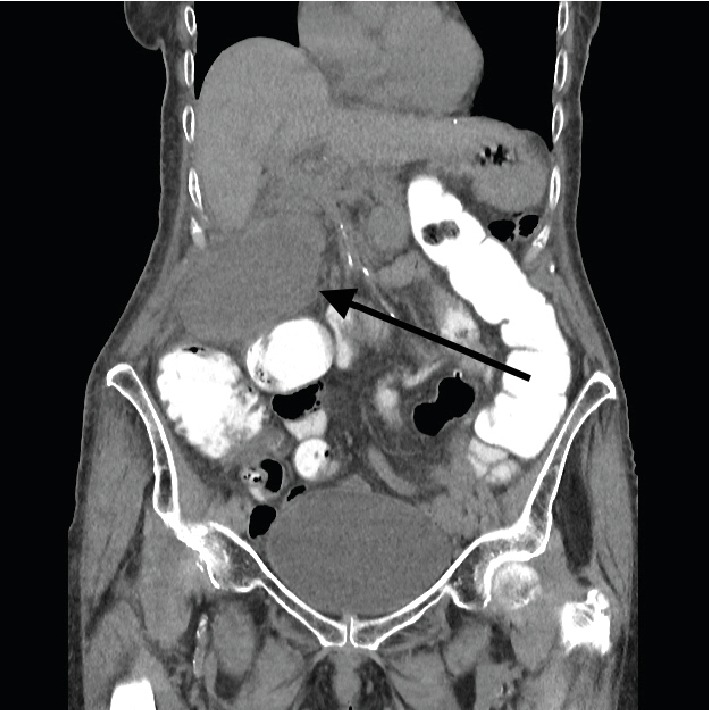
Coronal nonenhanced CT image showing a markedly distended gallbladder (black arrow) and fatty infiltration.
